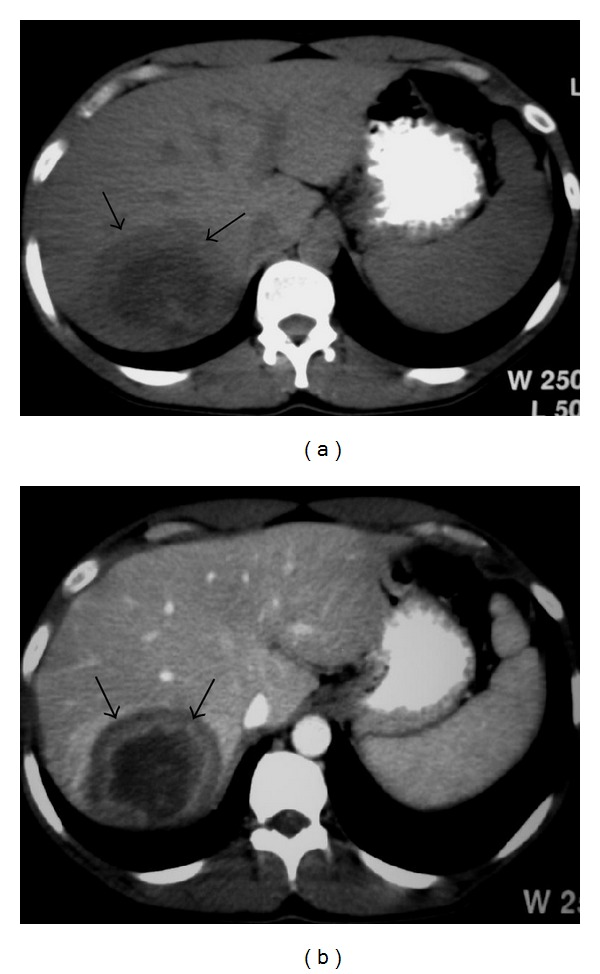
A 44-year-old man with NHL involving liver. (a) Nonenhanced axial CT of the liver reveals heterogeneous mass with central hypoattenuation (arrows). (b) Axial contrast-enhanced CT shows peripheral enhancement of the lesion (arrows).
